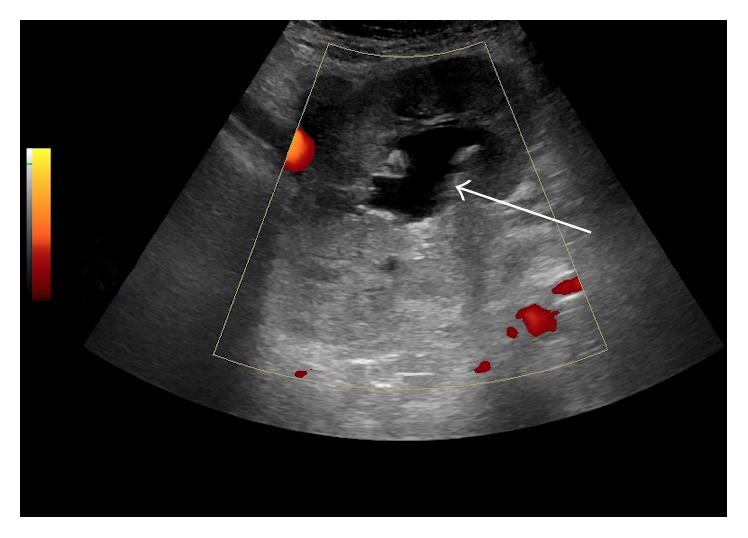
Power Doppler ultrasound demonstrates no internal vascularity within the enlarged hyperechoic left lower quadrant renal allograft raising concern for renal artery thrombosis and impending infarct. Note the moderate dilatation of the allograft collecting system (arrow).
